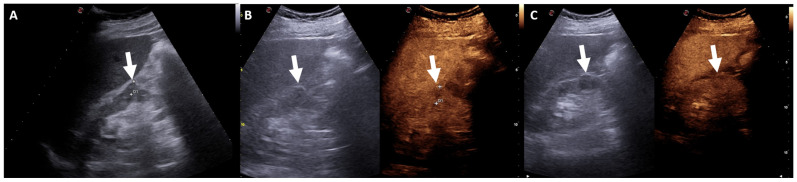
Renal ultrasound examination, B-mode (A) and CEUS scans (B,C). Small renal cortical lesion isoechoic to adjacent parenchyma (arrows). After an injection of intravenous contrast medium, the lesion shows enhancement.
